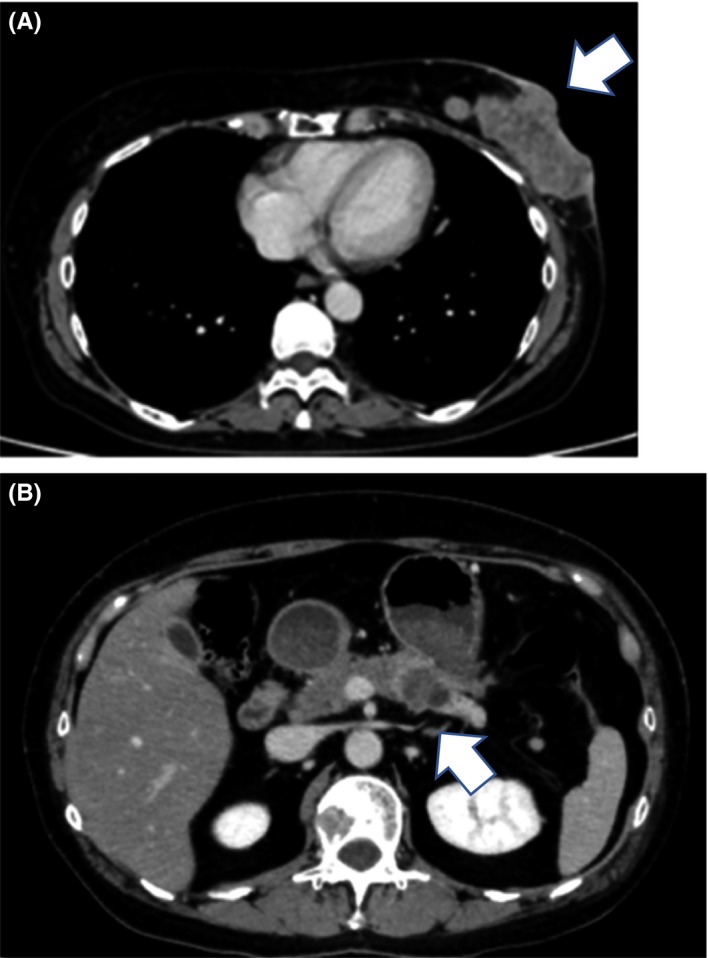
Computed tomography before treatment. A, A mass measuring 7 cm in the left breast with ulceration (Arrow). B, A mass measuring 2 cm in the tail of the pancreas (Arrow)
